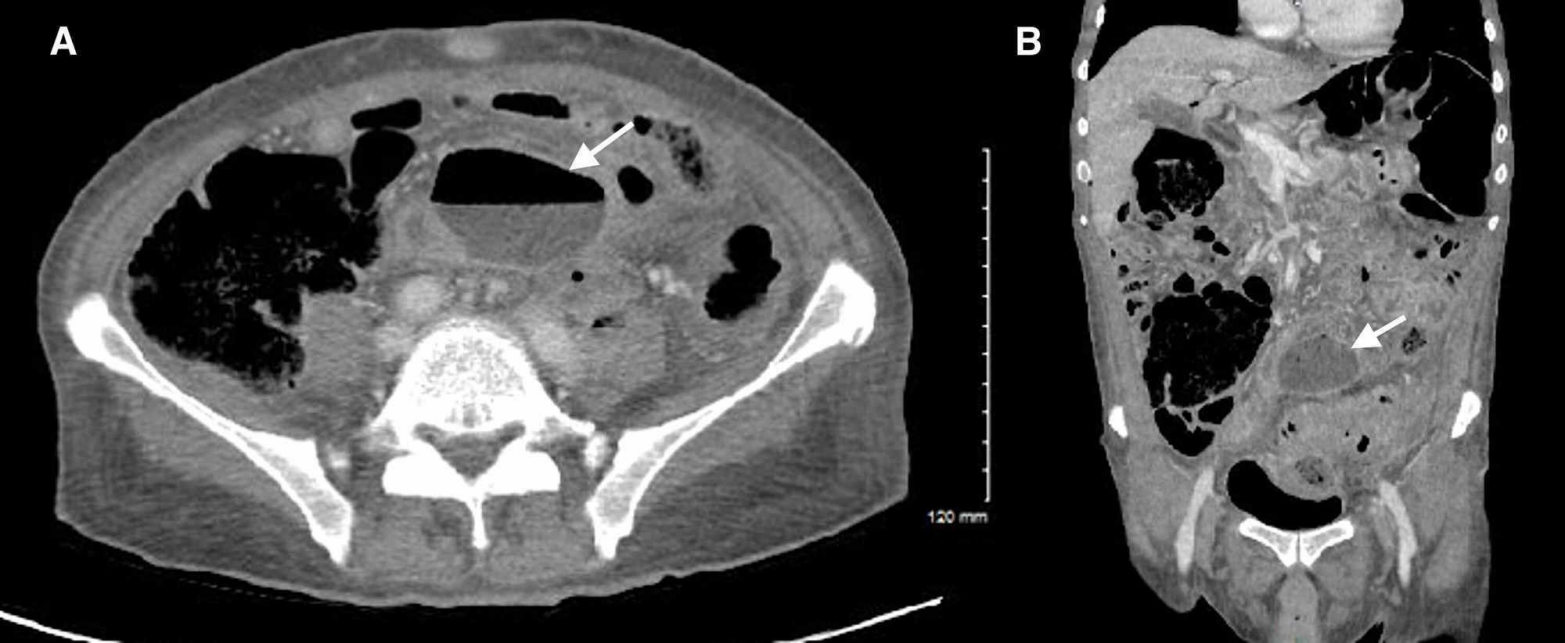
CT of the abdomen/pelvis demonstrating an intra-abdominal abscess collection #1 (white arrows) secondary to sigmoid colon perforation. Axial (A) and coronal (B) planes.
